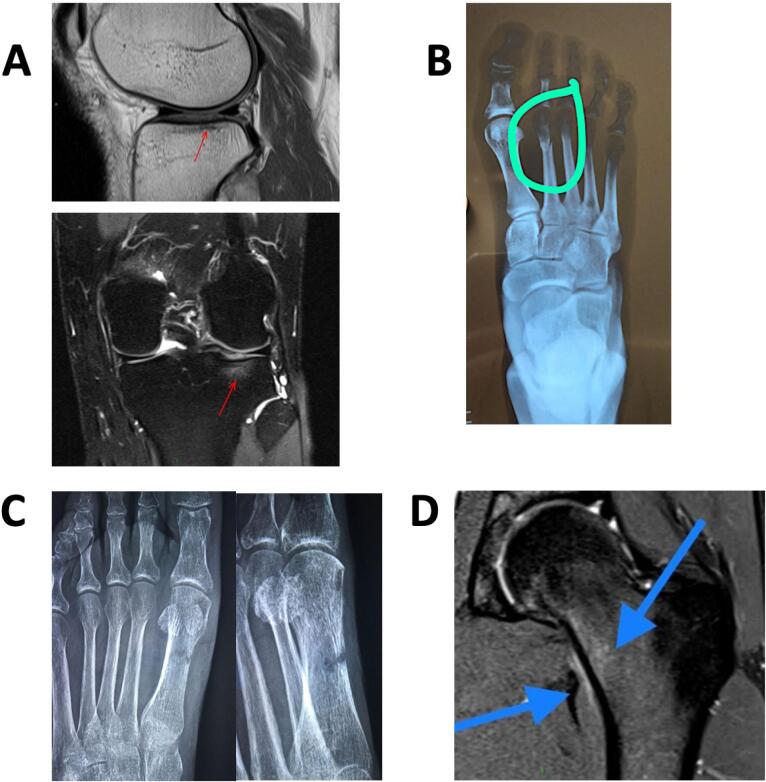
Images of stress fractures. A: 37-year-old male with knee pain after walking 5 km; B: 23-year-old female with oligomenorrhea, BMI of 16 kg/m^2^, pain after 10 km running; C: 28-year-old male after increased training for a running competition; D: Grade 1 SF based on MRI findings and using the Fredericson classification system (see Table 2) (33).
